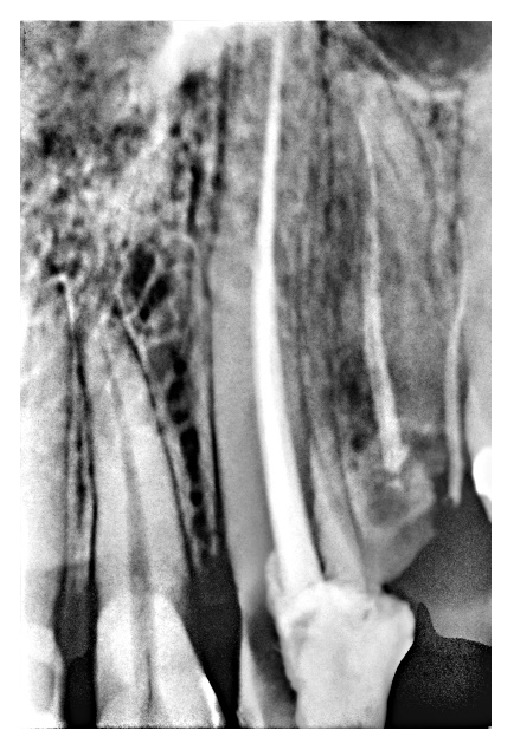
Postoperative radiograph obturated with gutta-percha and AH Plus Sealer.
